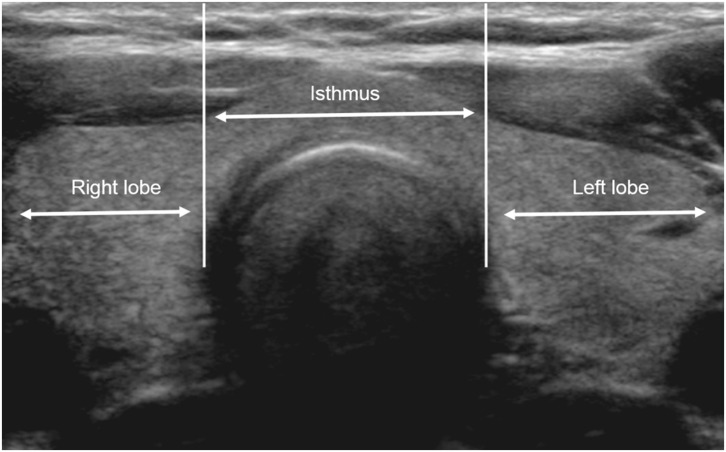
Boundary of the thyroid isthmus on ultrasonography (US). The lateral border of the isthmus is defined by two imaginary lines on transverse US images perpendicular to the skin surface from the most lateral tracheal wall. Thyroid nodules are classified as being of isthmic or lobar origin according to the location of its larger portion.
